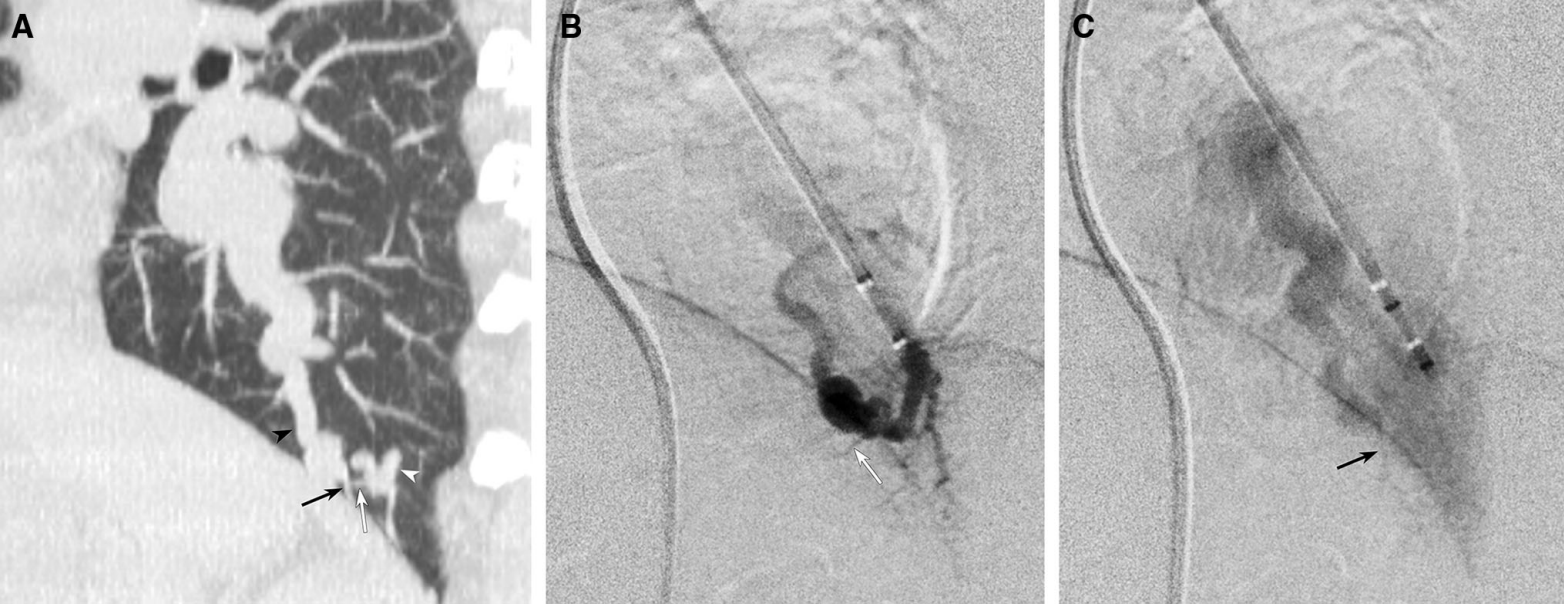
Definition of the last normal branch originating from the sac. A Oblique sagittal MPR CT image shows two branches originating from the sac. The direction of the proximal branch (white arrow) forms sharper angle to the feeding artery (white arrowhead) compared to the draining vein (black arrowhead). The distal branch (black arrow) forms very sharp angle with the draining vein (black arrowhead), but it forms obtuse angle to the feeding artery (white arrowhead). The proximal branch was classified as the last normal arterial branch, and the distal branch was classified as pulmonary vein due to the branching angle. B Lateral image of selective PAG during pulmonary arterial phase shows a pulmonary arterial branch (white arrow) from the sac which was detected on MDCT. C Lateral image of selective PAG during pulmonary venous phase shows a pulmonary venous branch into the sac (black arrow) which was detected on MDCT
